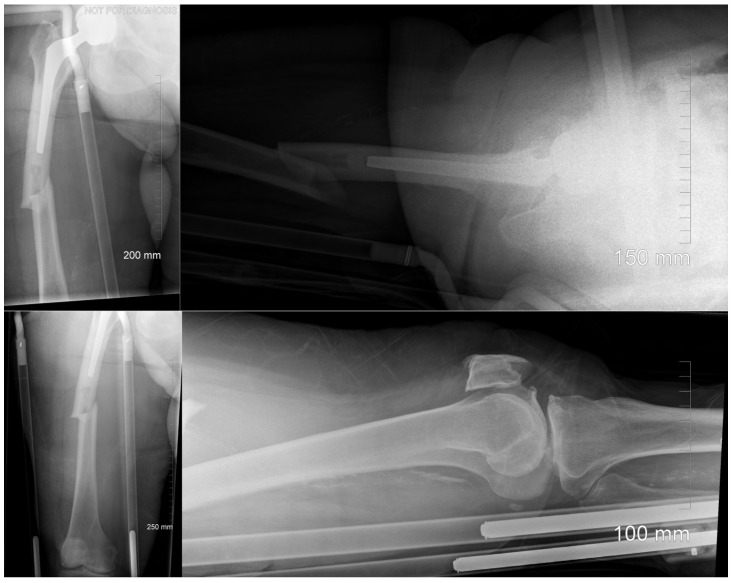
Radiographs demonstrating an acute atypical mid-shaft right periprosthetic femur fracture distal to the femoral stem (Vancouver Type C).
